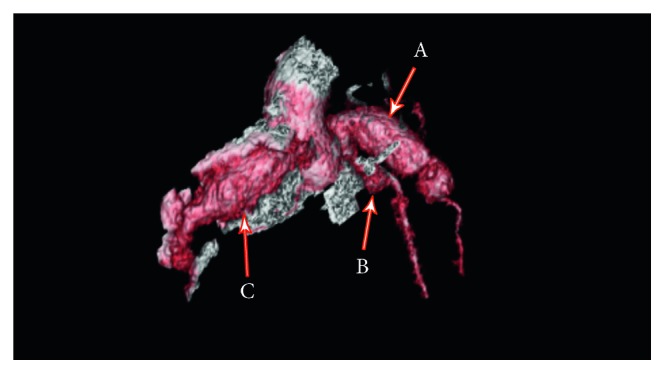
CT angiogram: there is a long fusiform aneurysm involving the proximal LAD measuring approximately 12 mm × 12 mm and extending over a length of 2.3 cm (A). The left circumflex is aneurysmal proximally measuring approximately 5 mm × 5.5 mm (B). There is a long fusiform aneurysm of right coronary artery measuring 16 mm × 16 mm and extending over a length of at least 3.2 cm (C).
